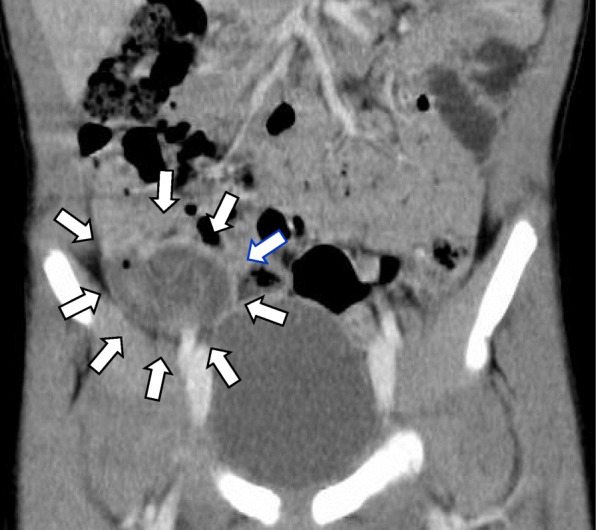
Computed Tomography scan of the abdomen. Swollen appendix (arrow) can be seen
